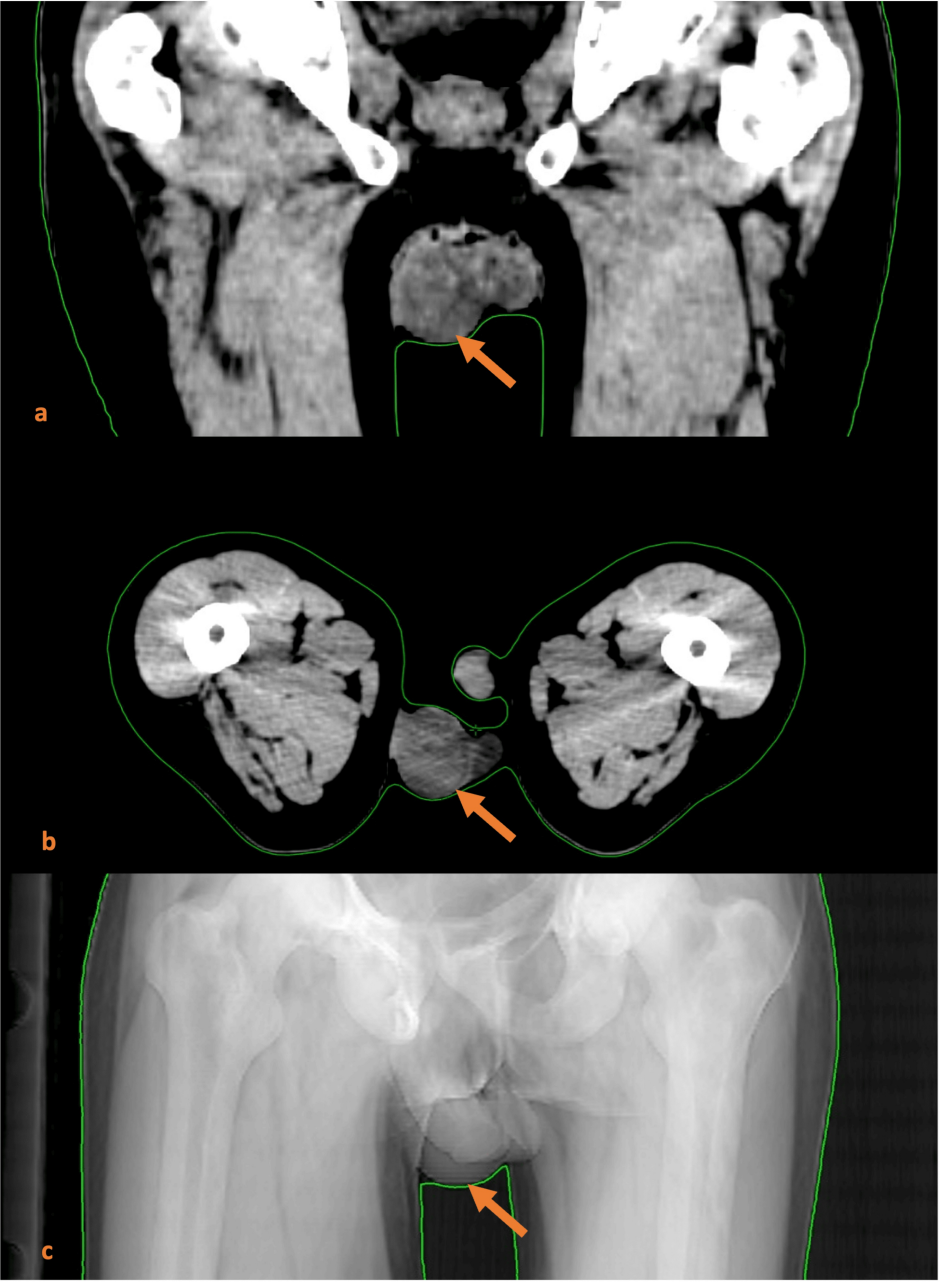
Dosimetric CT scan demonstrating the 41-mm solid heterogeneous testicular mass (orange arrow) (a) Coronal CT treatment planning slice showing a well-defined soft-tissue lesion centered within the right testis.
